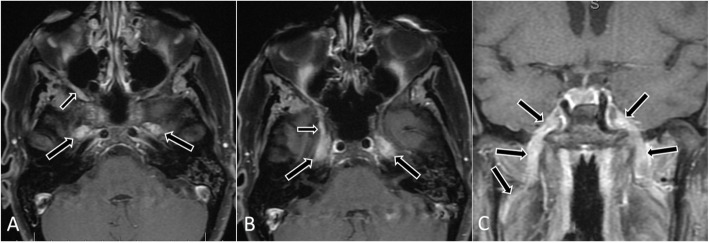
Postcontrast MR images of a 58-year-old-woman with relapsed Mantle cell lymphoma who presented with facial and jaw pain and parasthesias on the cheek from hematogenous spread (patient 16). a Axial image demonstrates involvement of bilateral mandibular nerves (V3) (large arrows) and the right maxillary nerve (V2) (small arrow). b Axial image demonstrates lymphoma in bilateral Meckle’s caves (large arrows) and the right foramen rotundum (V2) (small arrow). c Coronal images demonstrating involvement of bilateral mandibular nerves (V3) (arrows)
